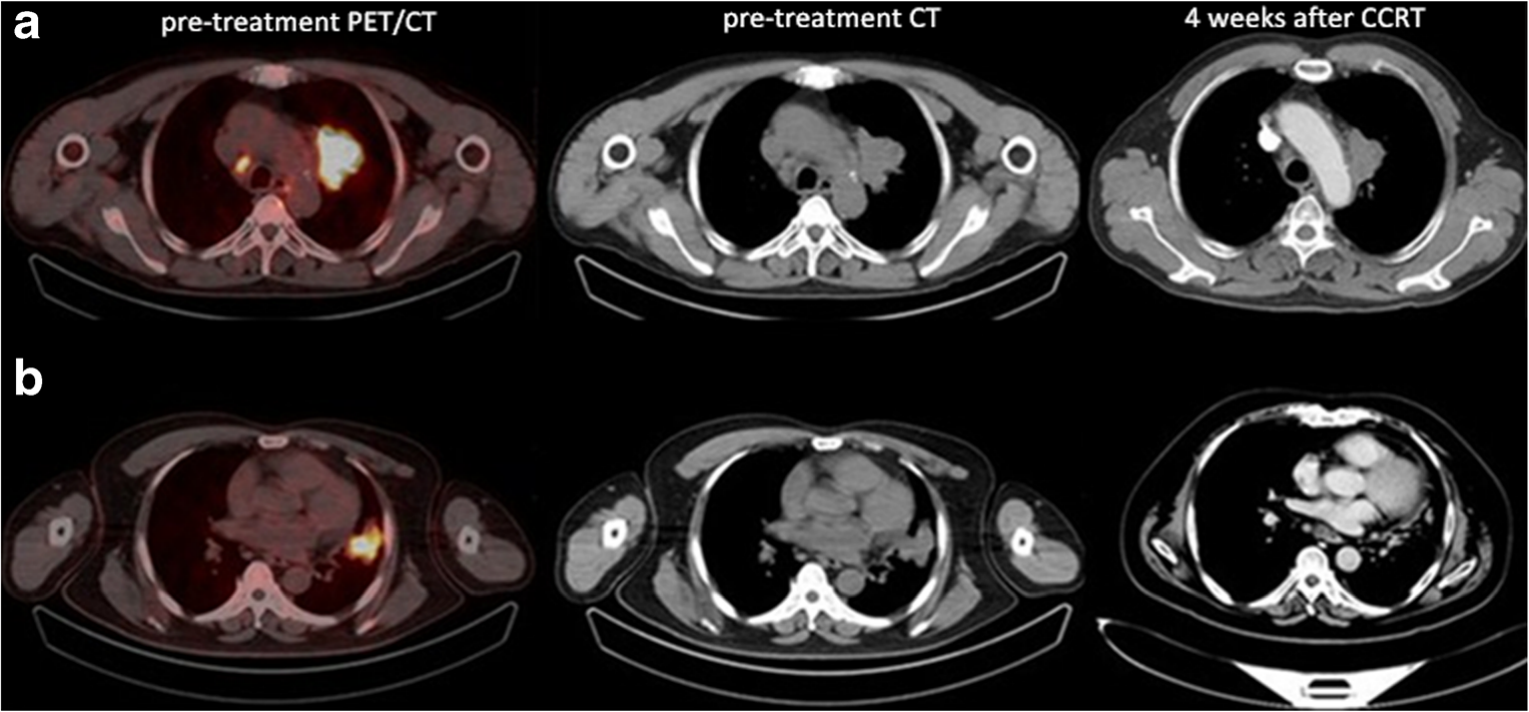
Two typical examples of ^18^F-alfatide PET/CT scans in patients with non-responding (a, T/NT_lung_ = 8.88) and responding (b, T/NT_lung_ = 6.47) tumors
